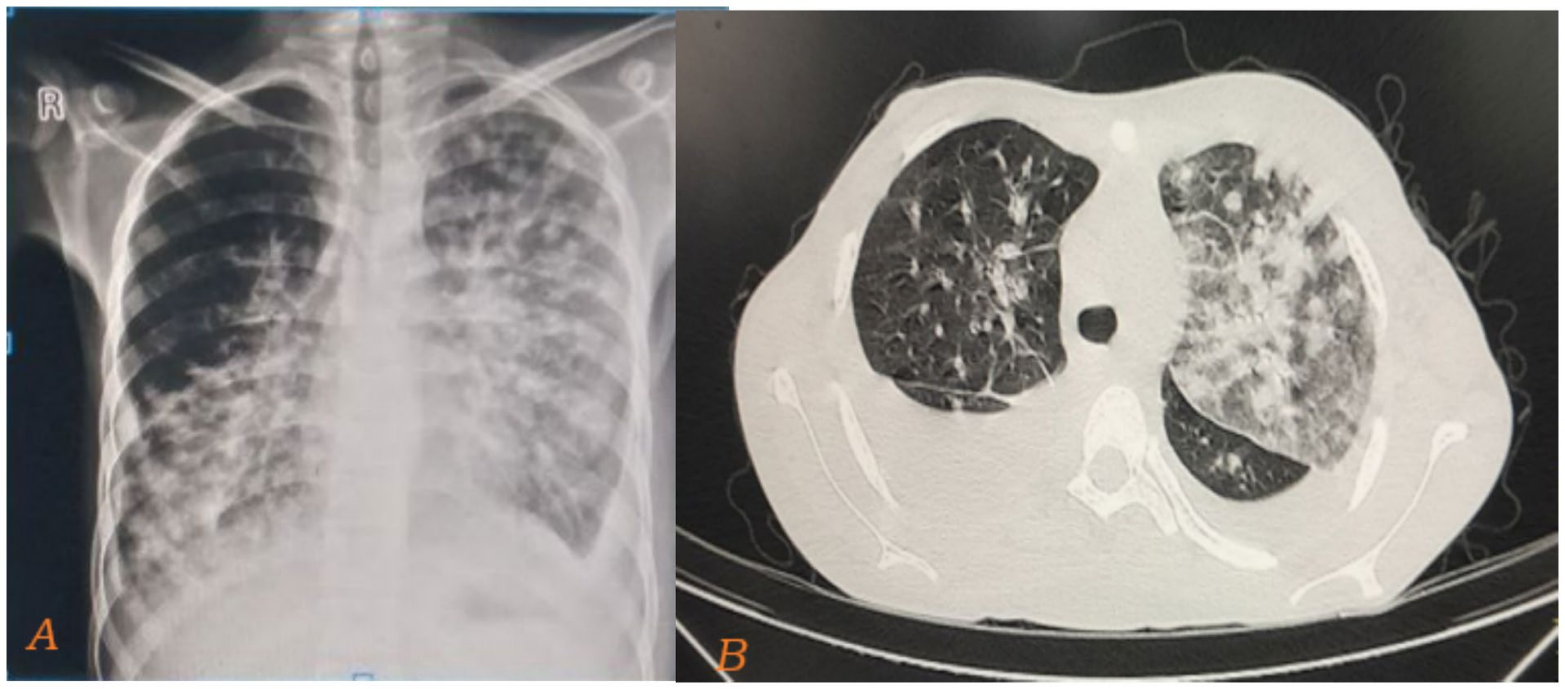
Taken during time of admission on august 21, 2024. Chest X-ray showing bilateral, diffuse, ill-defined, patchy opacities predominantly affecting the mid and lower lung zones (A). The cut slice of the CT scan of the same patient also reveals bilateral, asymmetric, peripherally dominant, ill-defined vascular nodules with a flame-shaped appearance around the bronchi and vessels. These nodules are accompanied by surrounding ground-glass and consolidative opacities, which are suggestive of Kaposi sarcoma (B).
